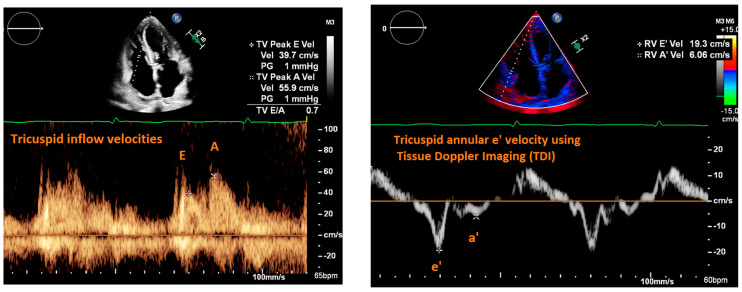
(Left): Tricuspid inflow velocities. Apical four chamber view. Pulse-wave Doppler sample volume is placed between the tricuspid valve leaflets at the level of the leaflet tips. Velocity tracing below. E wave corresponds to rapid right ventricular filling. A wave corresponds to the right atrial systole. (Right): Tricuspid annular velocity using tissue Doppler imaging. Tricuspid e’ corresponds to velocity of right ventricular tissue relaxation. Tricuspid E/e’ can be used to estimate RAP.
